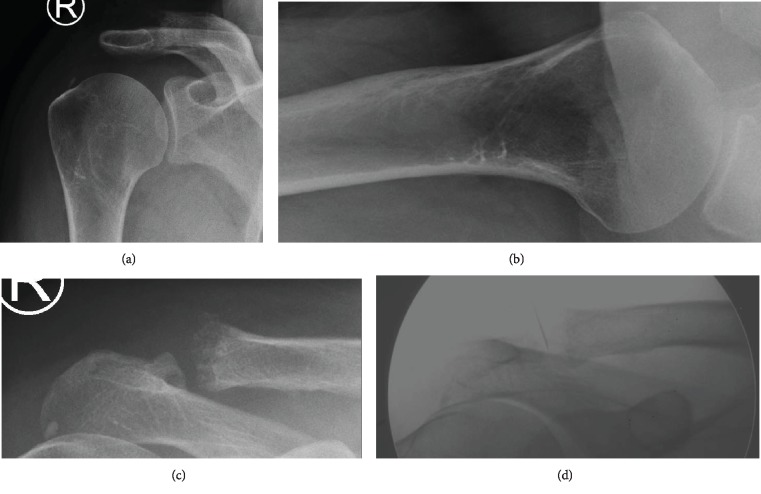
X-ray and fluoroscopic views of the right shoulder. (a) Anterior-posterior view. (b) Axial view. (c) Zanca view. (d) Acromioclavicular small-needle aspiration under fluoroscopic guidance.
